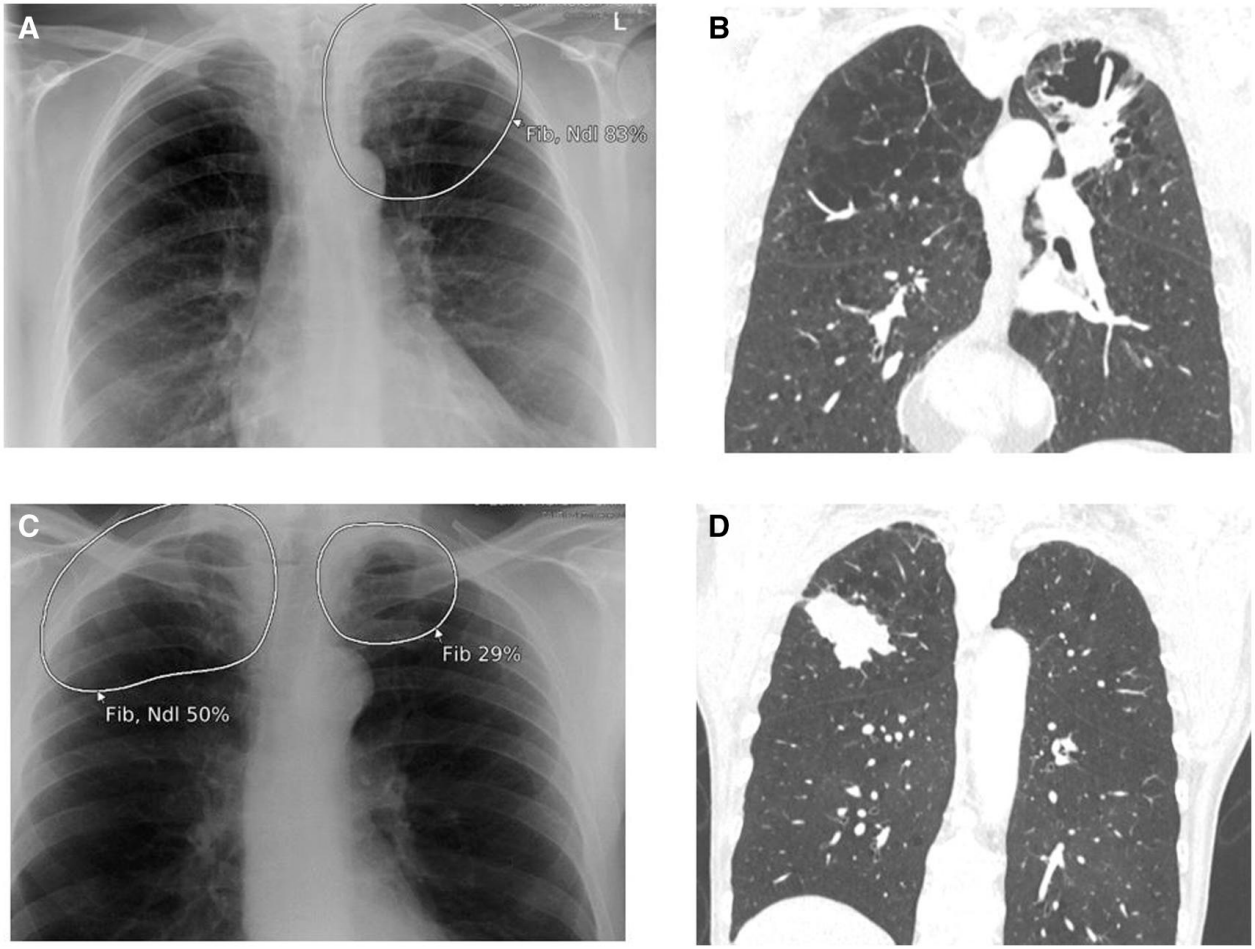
A 63-year-old female with no prior history of smoking, generally unwell, recovering from a previous chest infection, underwent a CXR (A) reported as NAD. The AI software demonstrates a suspicion of left apical fibrosis, with secondary suspicion of nodule (large white circle). Five years and 8 months later, the patient underwent a CT scan (B) as work-up for complex rheumatoid arthritis issues; this demonstrates the presence of a left apical tumour confirmed as stage IV LC. A 78-year-old male with a history of smoking and night sweats underwent a CXR (C) reported as NAD. The AI software demonstrates suspicion of bilateral apical fibrosis with secondary suspicion of a nodule on the right. Five years and 3 months later, the patient underwent a CT scan (D) due to cough and history of colon cancer, demonstrating a right upper lobe mass confirmed as stage 3 b LC. AI = artificial intelligence; CXR = chest radiograph; LC = lung cancer; NAD = no abnormility detected; SOB = shortness of breath.
